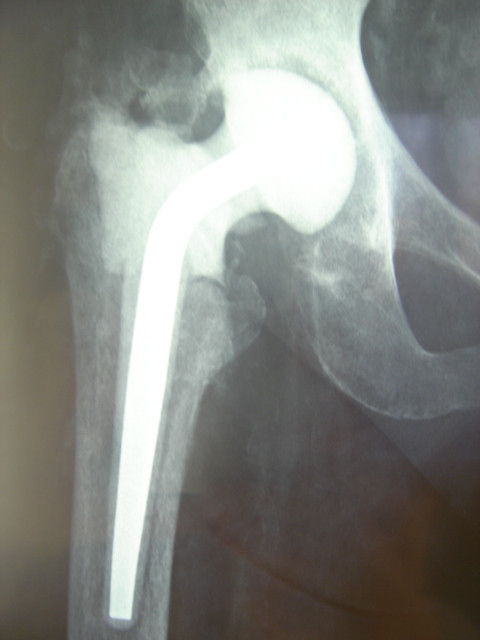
Clinical case (patient GF). Postoperative X-ray prior to hip spacer removal.
